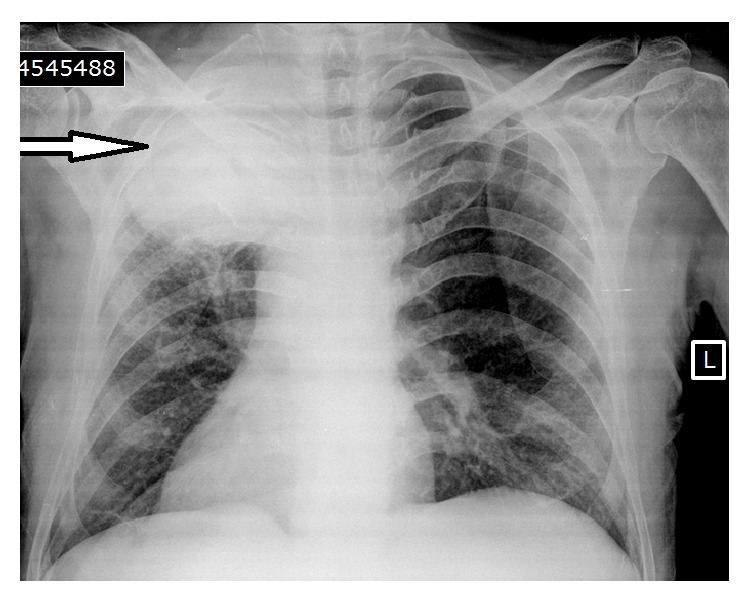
Chest X-ray image showing situs inversus and an abnormal 10 cm opacity at the right upper lung.
